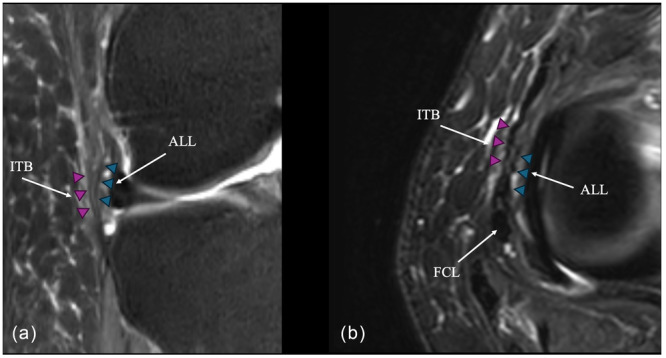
Coronal (a) and axial (b) reconstructions of T2‐weighted knee magnetic resonance imaging (MRI) scans of three Tesla. The anterolateral ligament (ALL‐blue arrows) is depicted, along with the iliotibial band (ITB‐purple arrows) and the fibular (lateral) collateral ligament (FCL).
